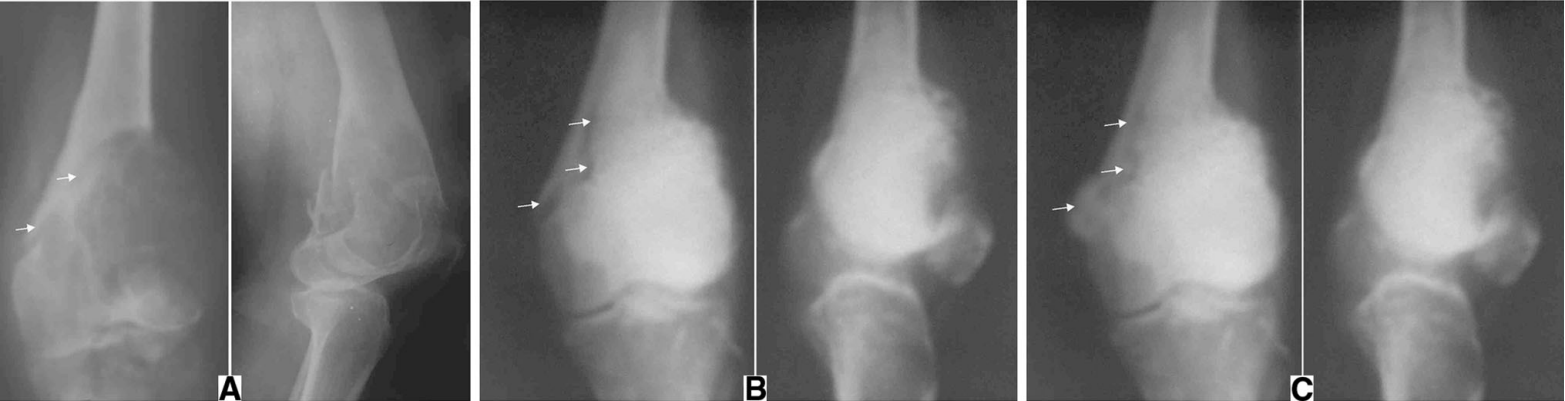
Radiographs of a 34-year-old male (case 1) showing a lesion in the distal end of the femur with a pathological fracture (a). Post-operative radiographs showing cementation (b). Sequential follow-up radiographs at 11 weeks showing good consolidation of the fracture line (c). (*Arrows* locating the pathological fracture)
